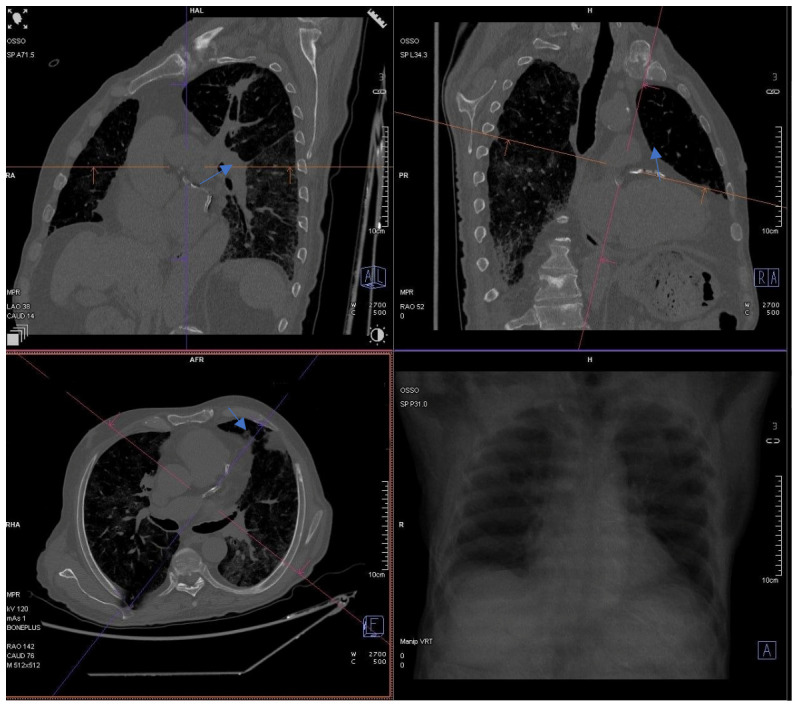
Non-contrast chest CT scans reveal calcific atherosclerosis of the LAD. The images show axial, coronal, and sagittal planes obtained from the LAD. Arrows in all panels indicate diffuse calcific atherosclerosis along the LAD.
